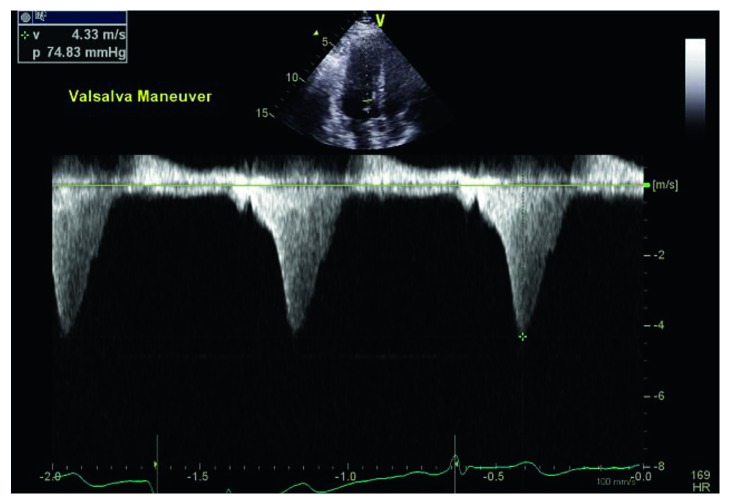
Echocardiogram showing 75 mmHg gradient in the left ventricular outflow tract with Valsalva.
